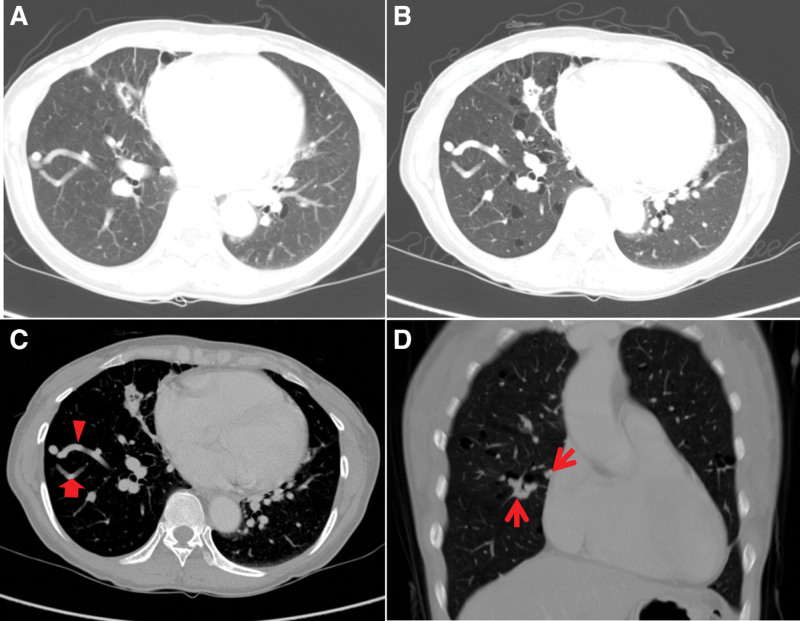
Initial chest CT image (A) and CT images after 3 years (B–D). (A) Initial chest CT image shows suspicion of AVM lesions in the RLL and small cystic lesions in both lungs. (B) Chest CT taken after 3 years reveals aggravating multiple cystic lesions and a persisting AVM lesion. (C) Chest axial CT image reveals multiple tubular lesions in the RLL and a feeding artery (red arrow) and draining vein (red arrowhead). (D) Another tubular enhancing lesion (red arrow) with the same finding as shown above are also identified in the right middle lobe on the coronal CT image. These findings are characteristic of pulmonary AVM. AVM = arteriovenous malformation, CT = computed tomography, RLL = right lower lobe.
